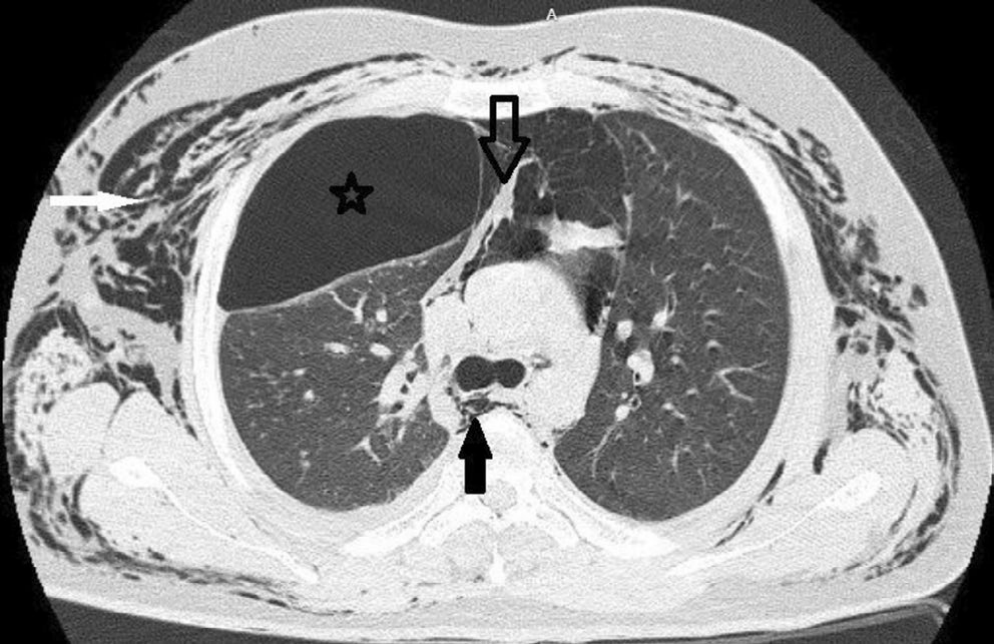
Soft tissue emphysema (white arrow), pneumothorax (asterisk), pneumomediastinum (black arrow), ruptured hydatid cyst (hollow arrow), in lung CT scan.
